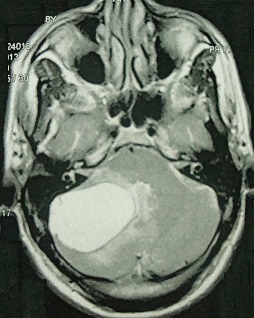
Brain MRI, T2-weighted axial sequence. High intensity signal. Simple cyst compressing the cerebellar vermis and fourth ventricle
